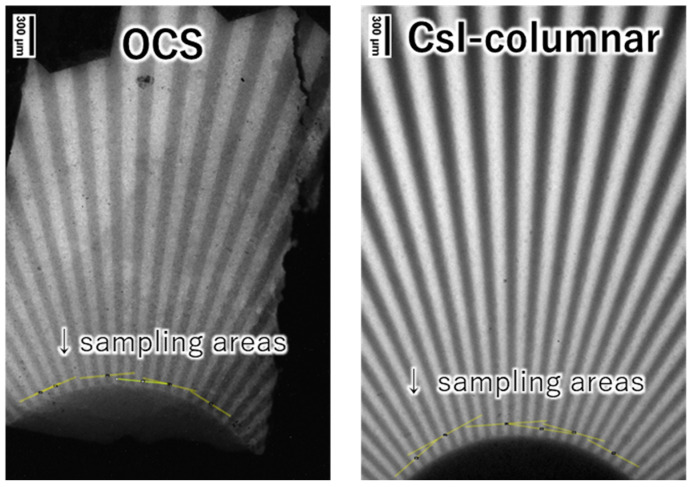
X-ray transmission images of the chart acquired using a 4× objective lens with a 100 ms exposure time: (left) OCS and (right) Tl:CsI columnar scintillator.
